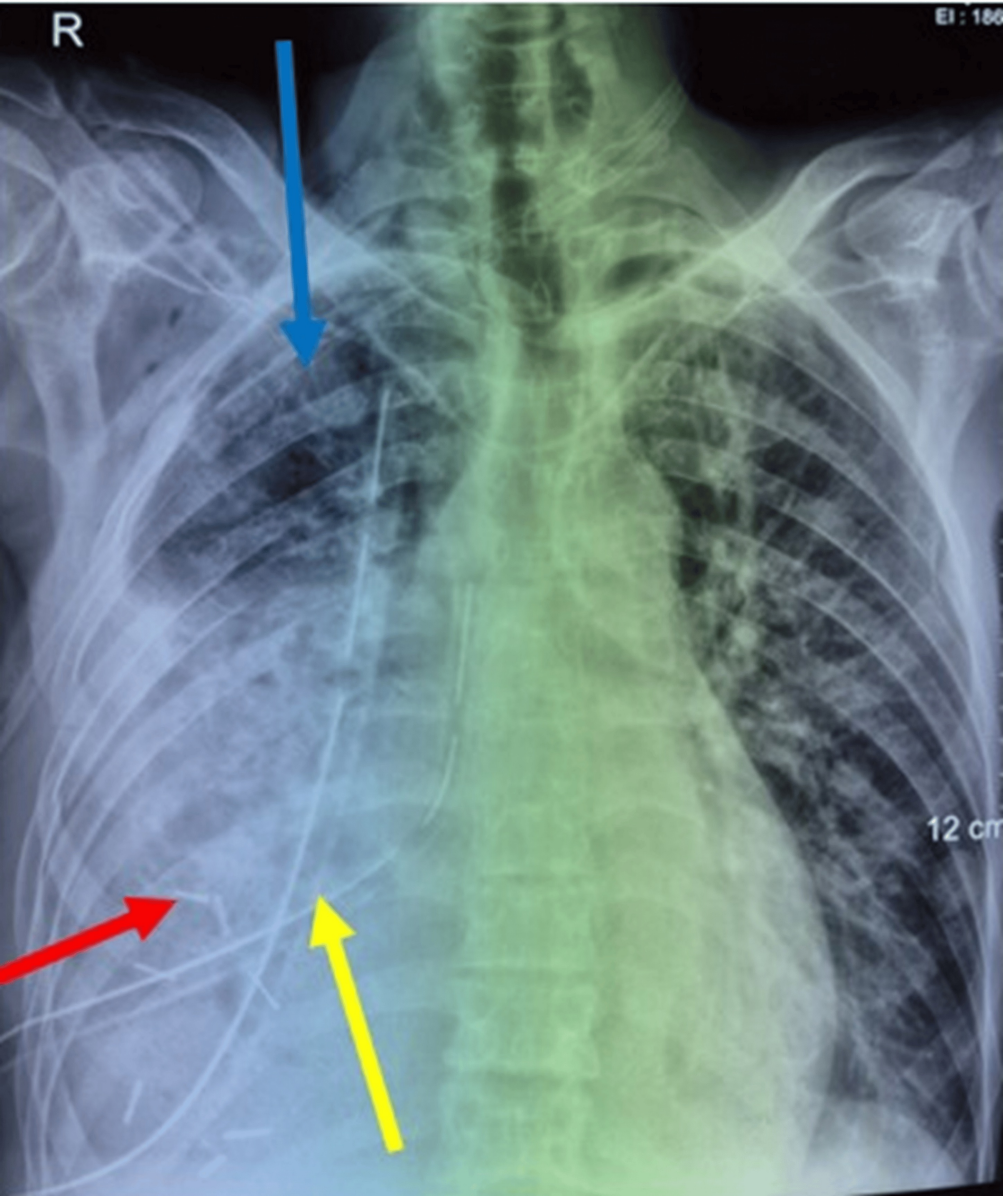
Chest X-ray. Revealed re-expansion of the lung adhered to the wall (blue arrow), with drains in place (yellow arrow) and clips on the tumor residue (red arrow).
